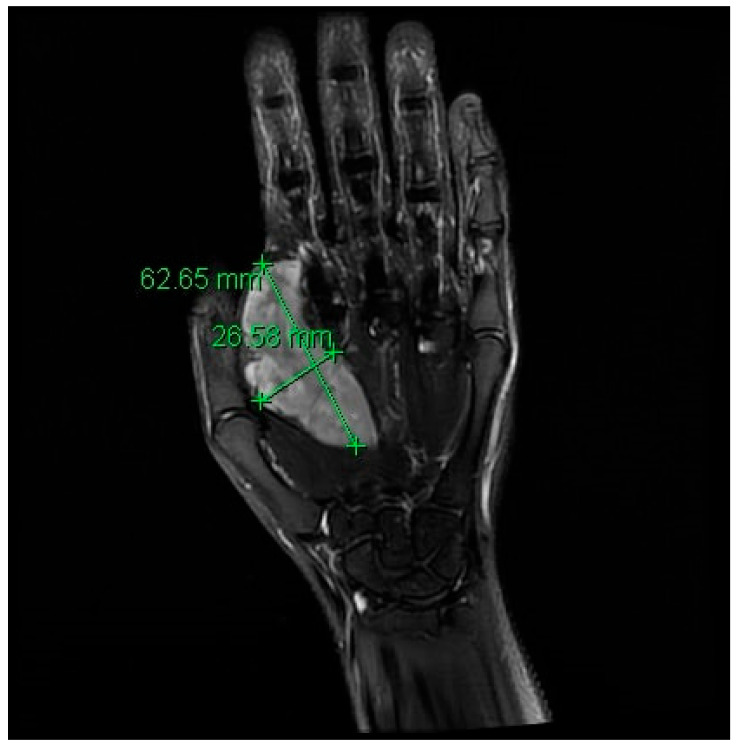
Magnetic resonance imaging revealing the 6.3 × 2.7 cm soft tissue sarcoma of the left hand.
